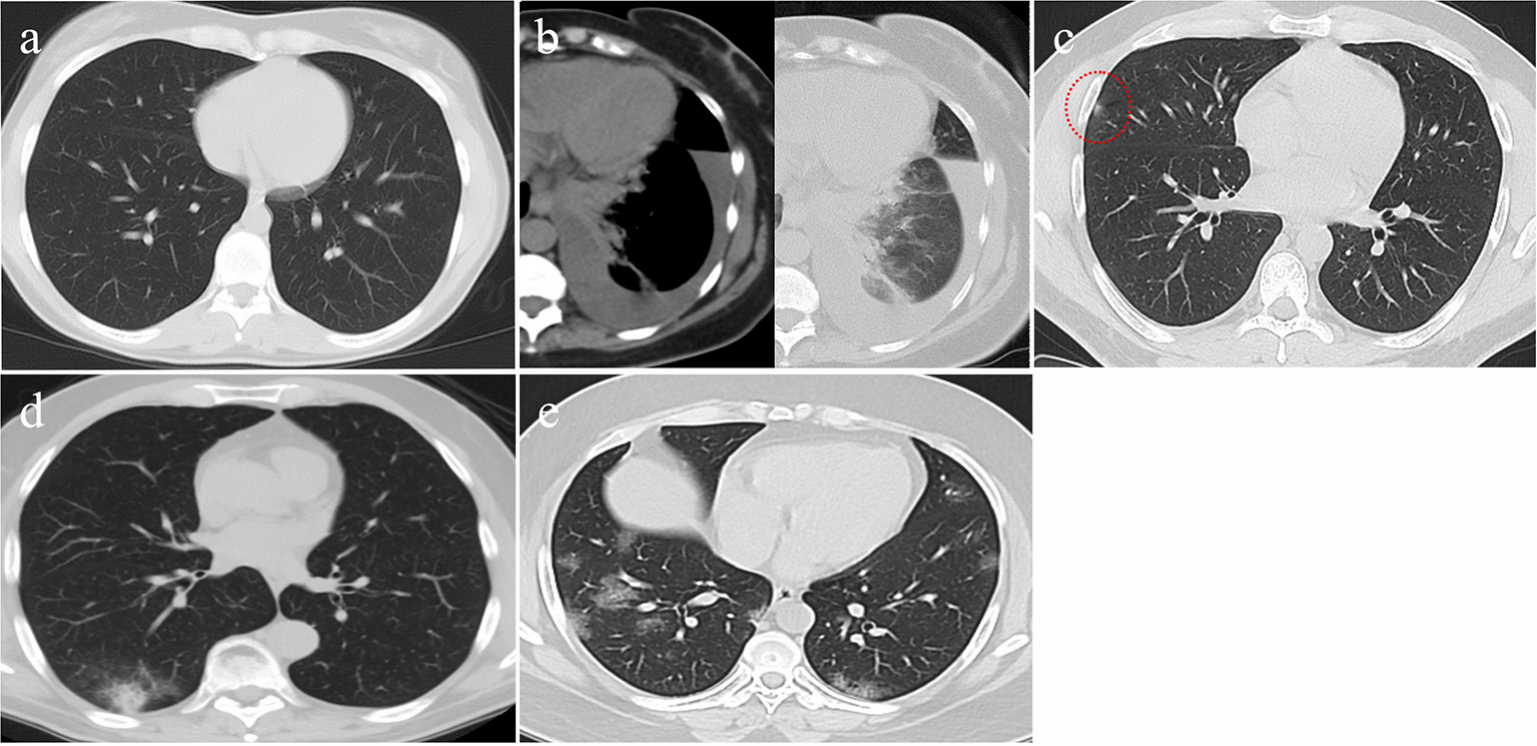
CT findings of different CO-RAD stages. (a) Normal CT findings, in keeping with CO-RAD1. (b) CT showed a left lower lobar patchy area of consolidation associated with moderate pleural effusion. This picture is suggestive of bacterial pneumonia (in keeping with CO-RAD2). Note that pleural effusion is very unlikely to occur with COVID-19 pneumonia. (c) A very tiny right middle lobar patchy area of faint ground glass opacity (red dashed circle), in keeping with CO-RAD3. (d) CT chest showed right lower lobar patchy area of ground glass veiling, mounting to consolidation, in keeping with CO-RADS 4. (e) Bilateral, mainly peripheral and lower lobar, small patchy areas of ground glass opacity, in keeping with CO-RADS 5.
